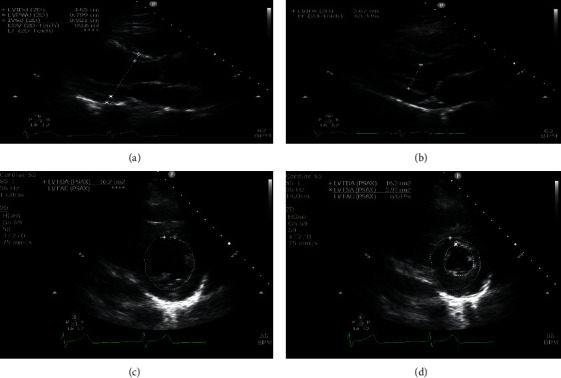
(a) Measurements of left ventricular chamber size and wall thickness dimensions in the parasternal long-axis view during diastole; (b) measurement of the left ventricular chamber size dimension in the parasternal long-axis view during systole. The left ventricular areas are measured at the midpapillary level of the left parasternal short-axis view during both diastole and systole; (c) left ventricular end-diastolic area; (d) left ventricular end-systolic area. LVIDd: left ventricular internal diameter diastole; LVPWd: left ventricular posterior wall diastole; IVSd: interventricular septum diastole; EDV: end-diastolic volume; EF: ejection fraction; LVIDs: left ventricular internal diameter systole; LVEDA: left ventricular end-diastolic area; LVFAC: left ventricular fractional area of change; LVESA: left ventricular end-systolic area.
